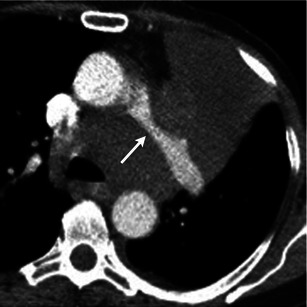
Contrast-enhanced axial CT image in a patient with left hilar lung cancer demonstrates the left main pulmonary artery being completely encased and narrowed by the left upper lobe mass (*arrow*), which also extends into the mediastinum
